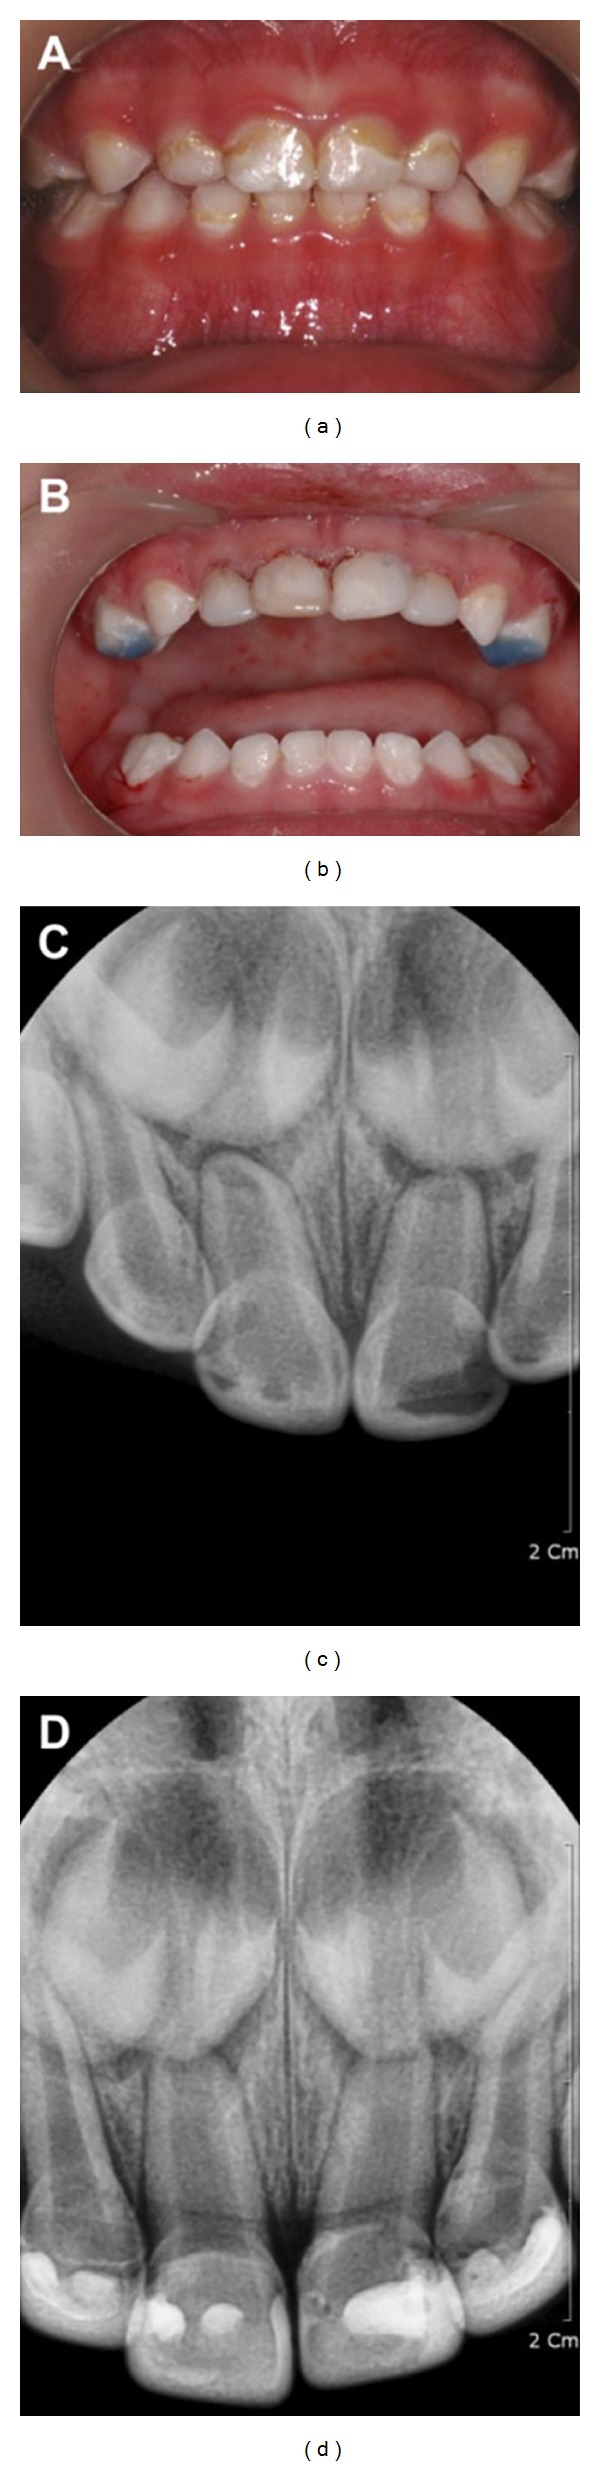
(a) Oral status of the patient before treatment and (b) restoration with RelyX Unicem. In order to avoid anterior teeth biting, 54 and 64 teeth were restored with blue resin. (c) Radiograph of the patient before treatment and (d) radiograph of the patient after treatment.
